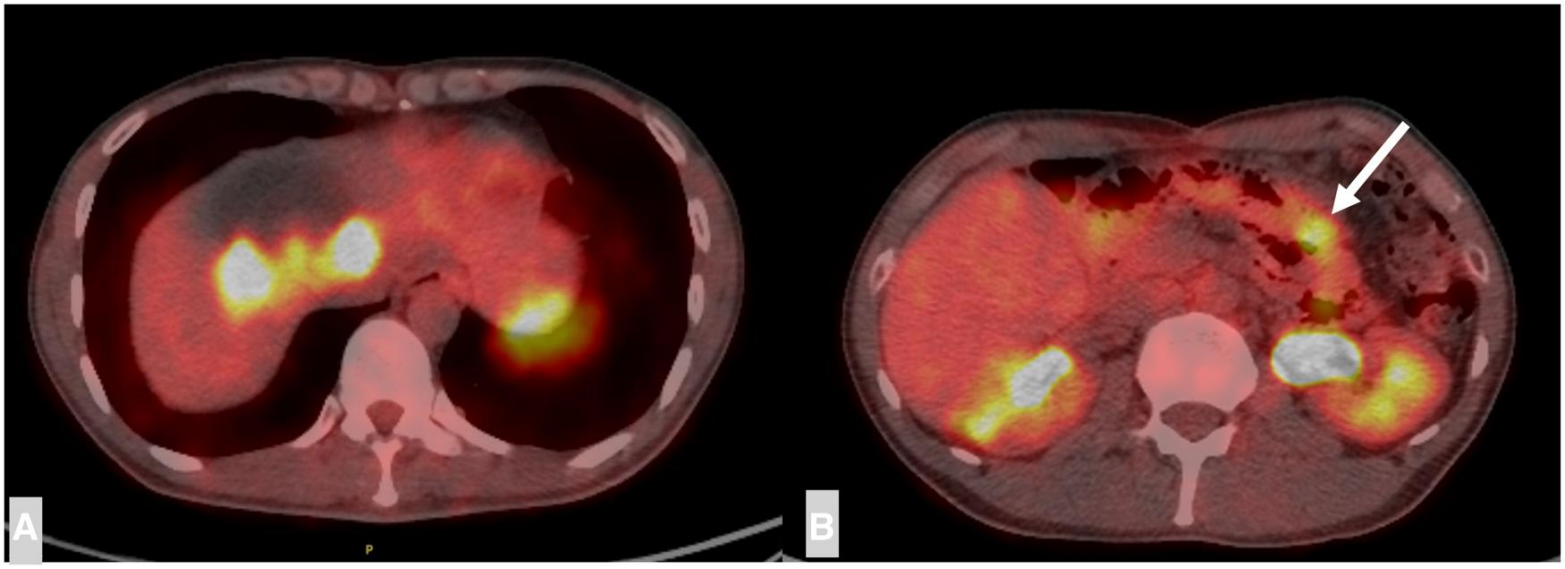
FDG-PET/CT of the axial image shows (A) FDG accumulation consistent with the solid components within the hepatic cyst and (B) FDG accumulation are also observed in a part of the small intestine (white arrow). Abbreviations: FDG = 18F-fluorodeoxyglucose; PET = positron emission tomography; CT = computed tomography
